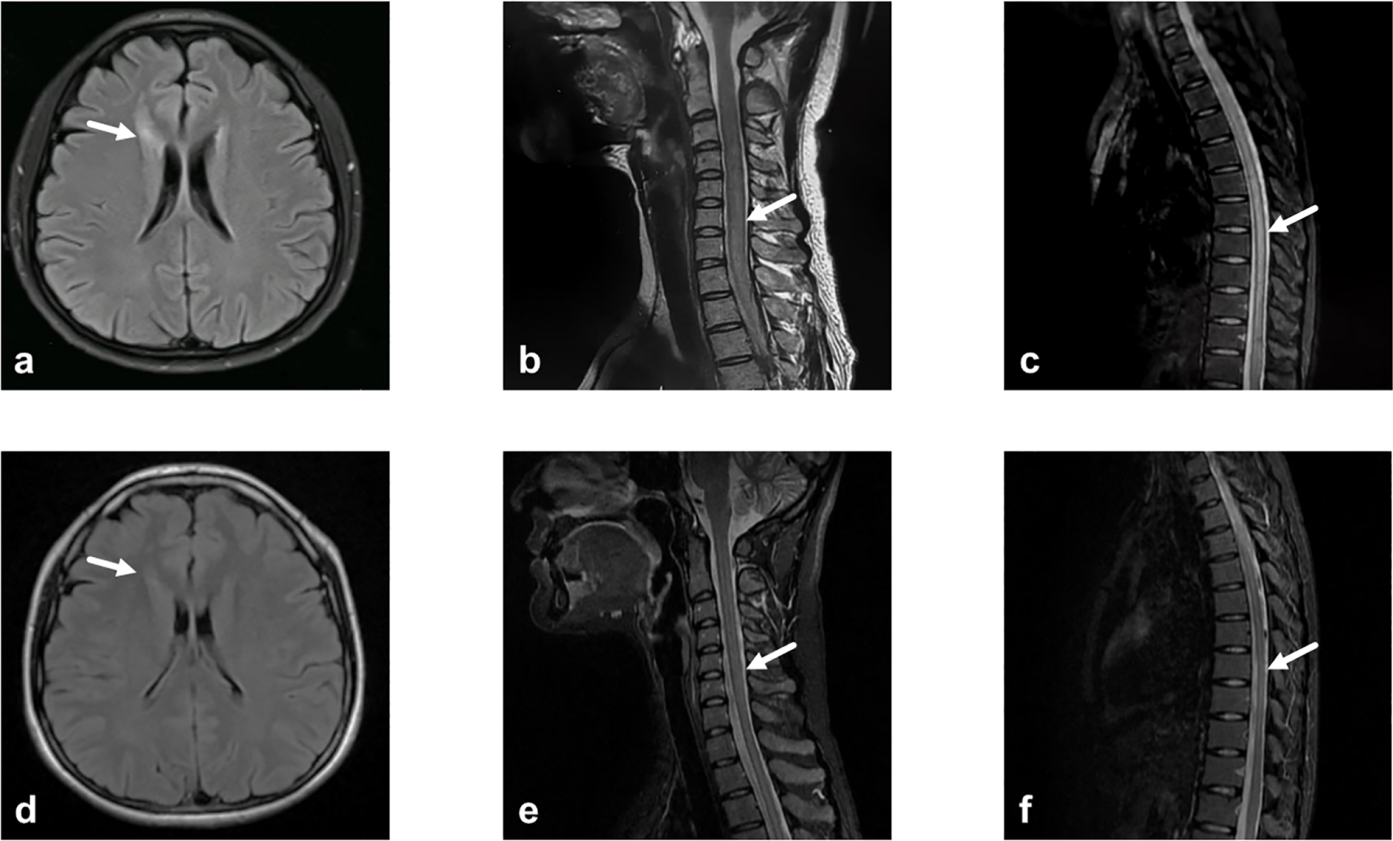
Imaging changes before and after ofatumumab treatment for case 2. (a) Axial T2-FLAIR brain MRI. A patchy FLAIR hyperintensity lesion is observed in the white matter adjacent to the right frontal horn of the lateral ventricle. (b) Sagittal T2-weighted cervical spinal MRI. The lower cervical spinal cord is swollen with diffuse T2-weighted hyperintensity, consistent with myelitis of the lower cervical cord. Long-segment hyperintensity spanning ≥3 vertebral segments is consistent with LETM. (c) Sagittal T2-weighted thoracic spinal MRI. The thoracic spinal cord is swollen with diffuse T2-weighted hyperintensity, consistent with thoracic myelitis. Long-segment hyperintensity spanning ≥3 vertebral segments is observed. (d) Axial T2-FLAIR brain MRI. Following OFA treatment, the lesion in the white matter adjacent to the right frontal horn of the lateral ventricle has completely resolved. (e) Sagittal T2-weighted cervical spinal MRI. After OFA treatment, a patchy T2-weighted hyperintensity is visible within the cervical spinal cord, showing significant reduction in extent and marked improvement of edema compared to previous imaging. (f) Sagittal T2-weighted thoracic spinal MRI. After OFA treatment, a patchy T2-weighted hyperintensity is visible within the thoracic spinal cord, showing significant reduction in extent and improvement of edema compared to previous imaging. FLAIR, fluid-attenuated inversion recovery; LETM, longitudinal extensive transverse myelitis; OFA, Ofatumumab.
